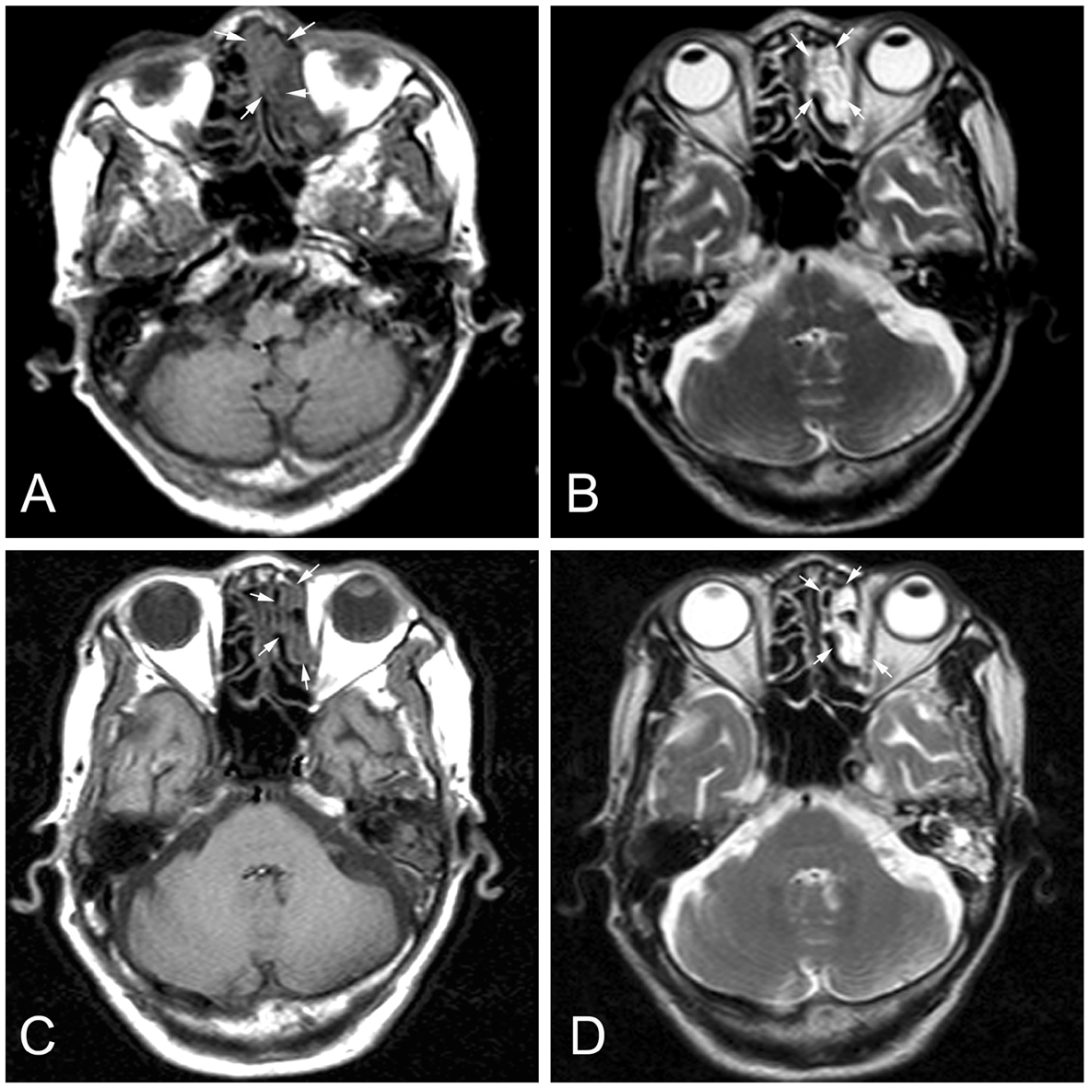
Radiological findings of the intranasal mass (A) Axial CT scan (T1) revealed that an irregular mass presented in the left nasal cavity displacing the nasal septum (white arrow). (B) CT scan (T2) showed that the mass also extended into left ethmoid sinus, but did not erode the bony margins of the medial wall of the left orbit (white arrow). After 6-month period of follow-up, axial CT scan T1 (C) and T2 (D) showed that location and size of the mass did not change remarkably (white arrow).
